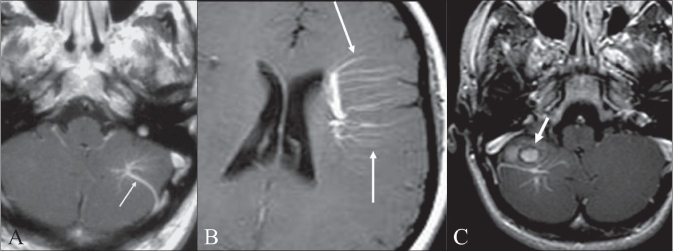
Medusa head sign. Postcontrast axial T1W MRI image of the brain (A) shows a evelopmental venous anomaly (arrow), with multiple, small, radiating veins forming a ‘Medusa head’ in the left cerebellar hemisphere. Postcontrast axial T1W MRI image of the supratentorial brain (B) shows a large developmental venous anomaly with multiple radiating veins (arrows) draining into it. Postcontrast axial T1W image of the brain (C) shows a large developmental venous anomaly and a round hyperintense lesion with a dark rim (arrow), suggestive of a cavernoma, anterior to it
